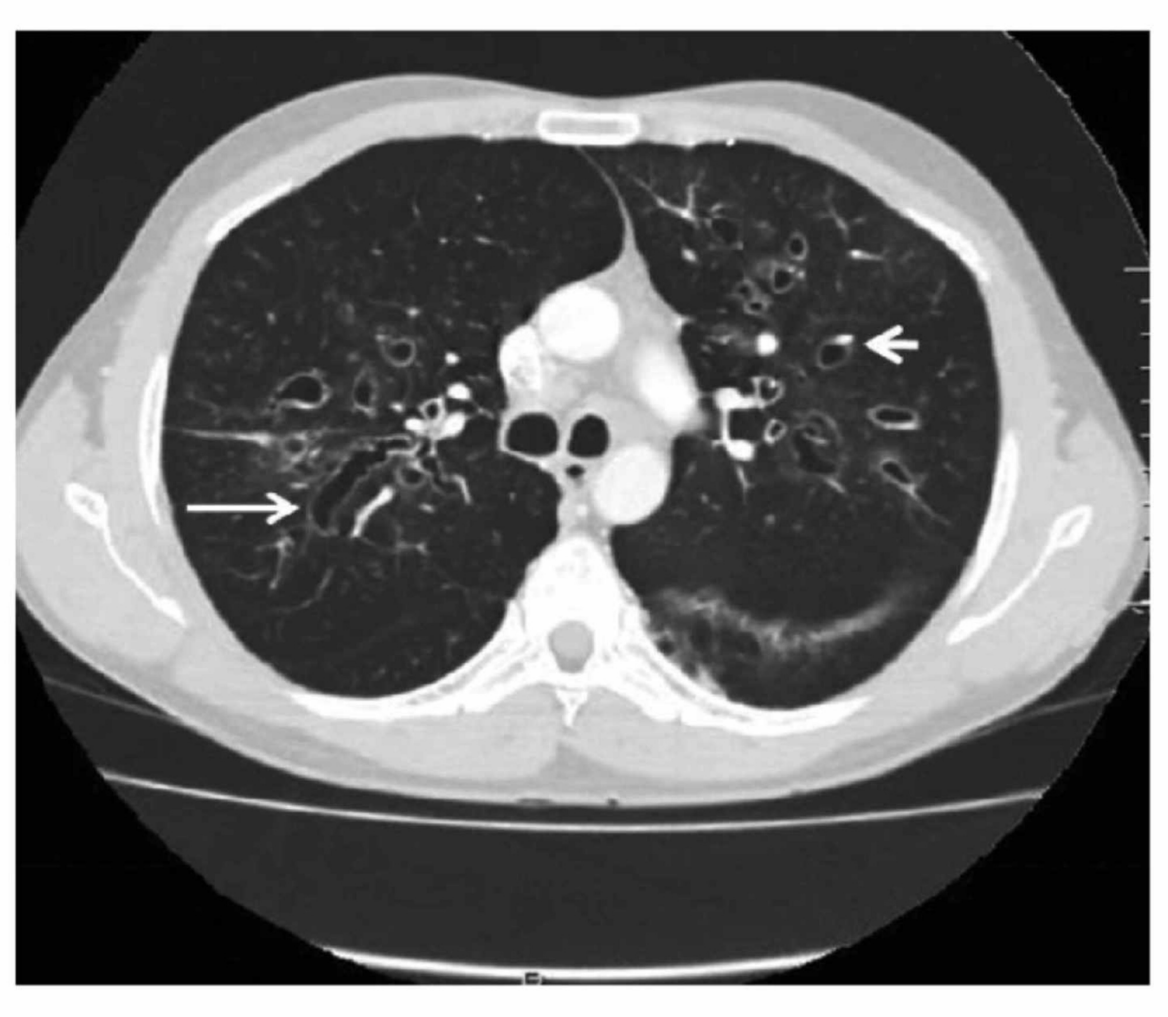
Computed Tomography (CT) of the Thorax CT showing ‘signet ring’ (short, thick arrow) and ‘string of pearls’ (long, thin arrow) appearances, indicative of central bronchiectasis. Mucoid impaction and dilated bronchi are also seen [3].
